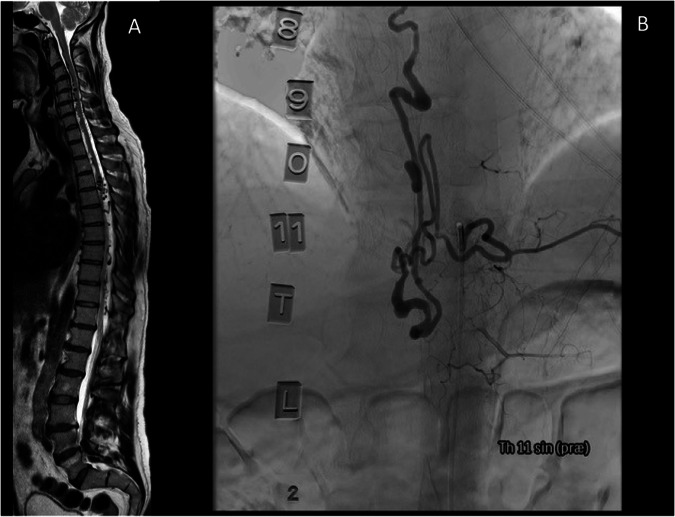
Magnetic resonance imaging (MRI) and angiography were performed prior to embolization of spinal arteriovenous malformation in our patient. Panel (A) shows an MRI sagittal image of the spinal column with multiple loci of tortuous vessels, particularly at levels Th4-Th6 and Th8-11. Image (B) of the panel shows the angiography performed prior to embolization.
